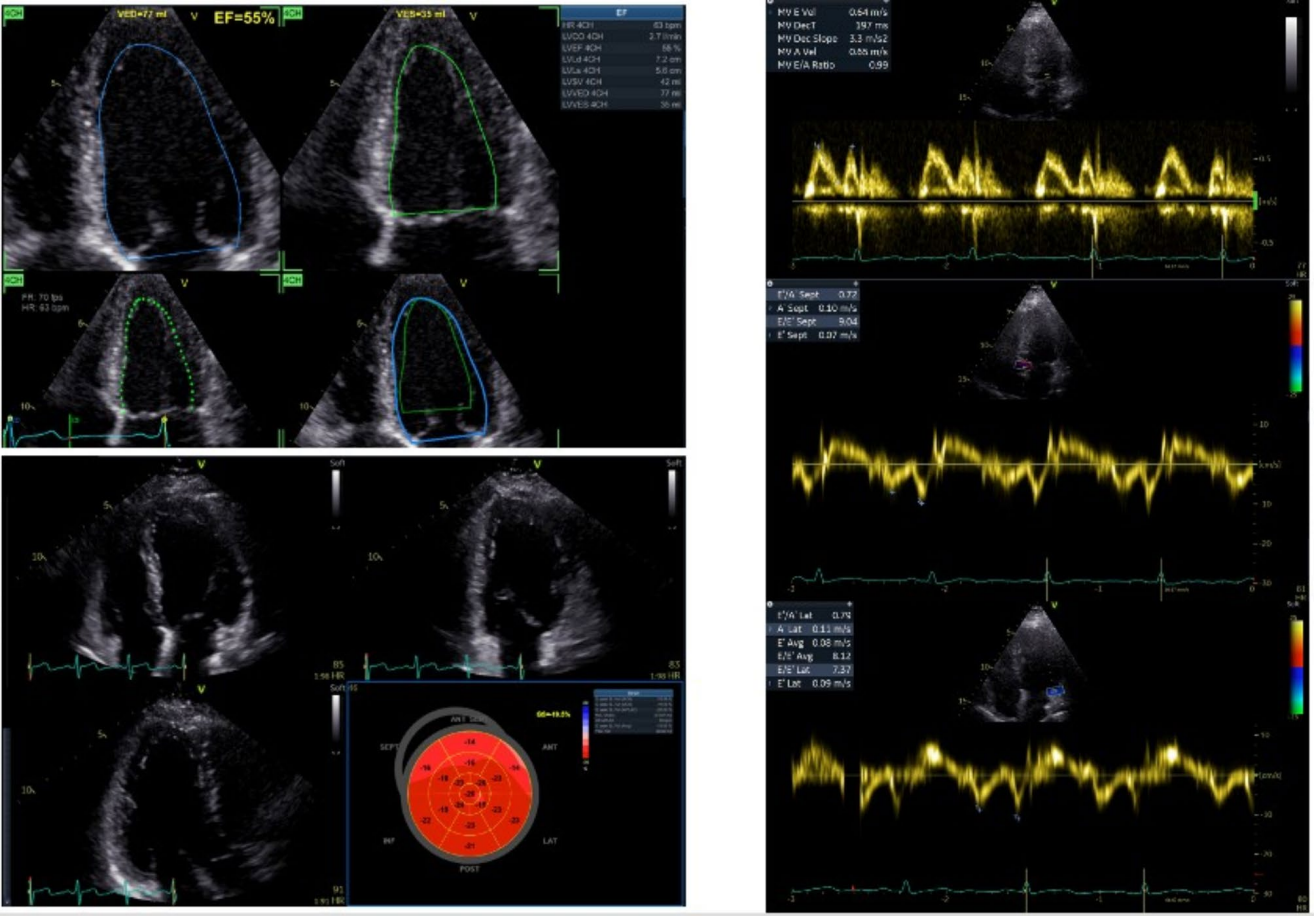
Representative images of echocardiographic acquisition.
